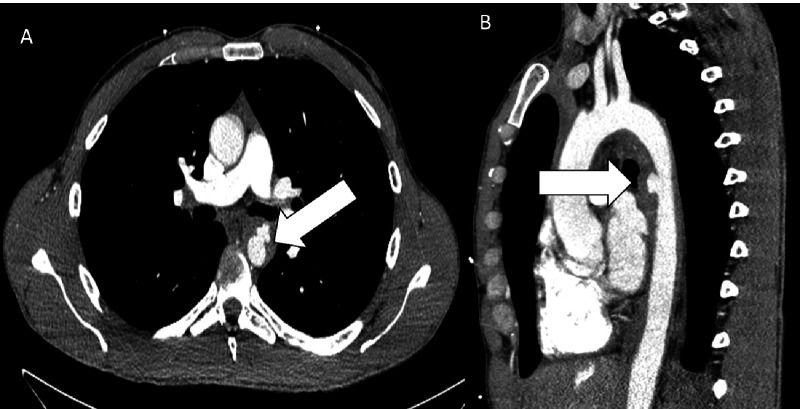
Computed tomography (CT) of the mid-chest A) Axial contrast-enhanced CT of the mid-chest showing dilation of the descending aorta with an intramural hematoma and penetrating ulcer (arrow) in the proximal descending aorta. B) Sagittal oblique CT showing the intramural hematoma and penetrating ulcer (arrow).
